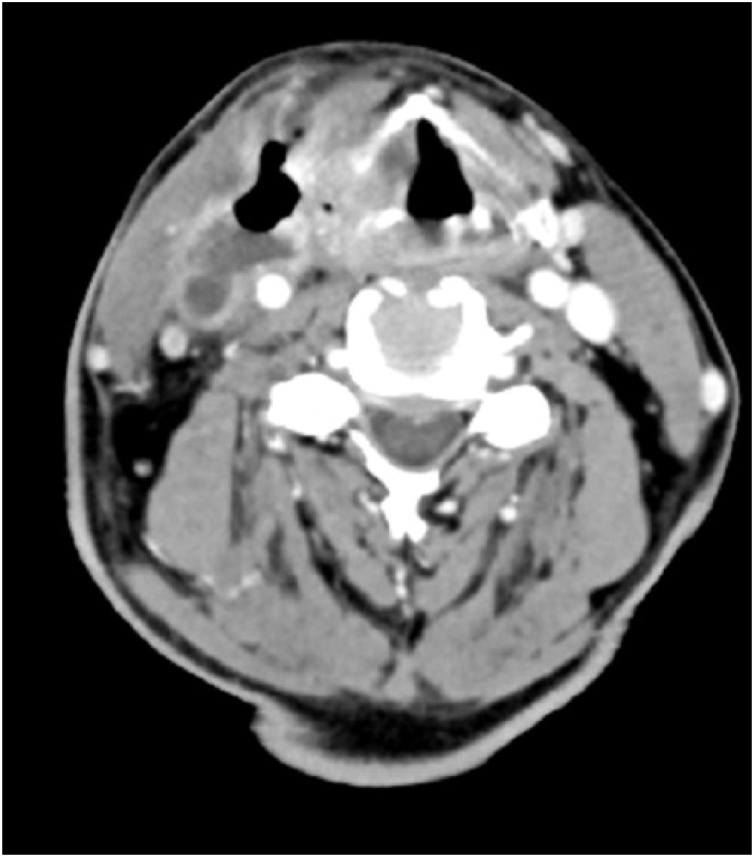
Axial CT scan showing direct spread of tumor through to hypopharyngeal wall and invasion of internal jugular vein.
